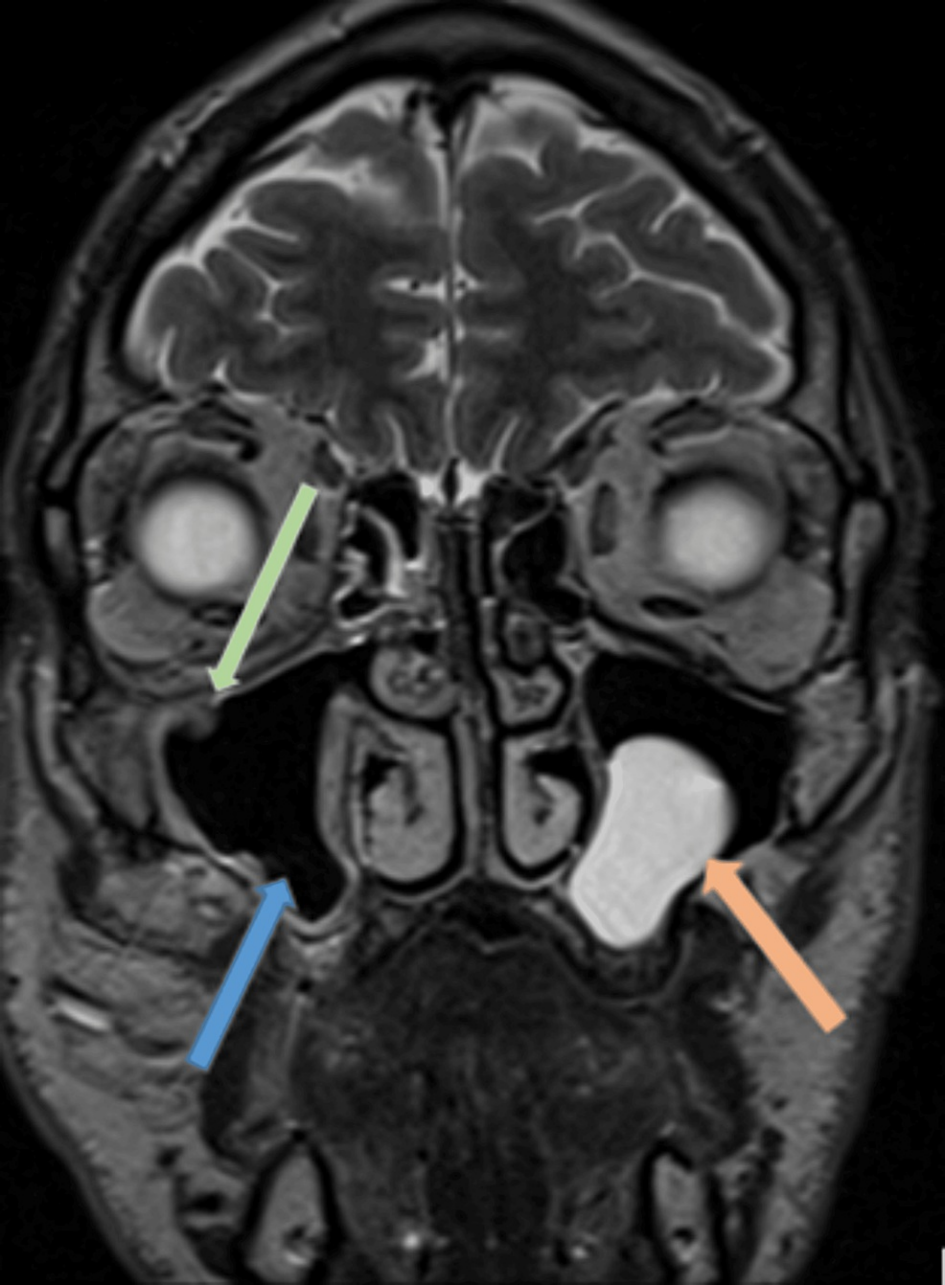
T2-weighted MRI – coronal image Significant reduction in right maxillary sinus opacification (blue arrow). The ION is visible within the maxillary sinus (green arrow). Stable polypoidal mucosal thickening in the left maxillary antrum (orange arrow).
